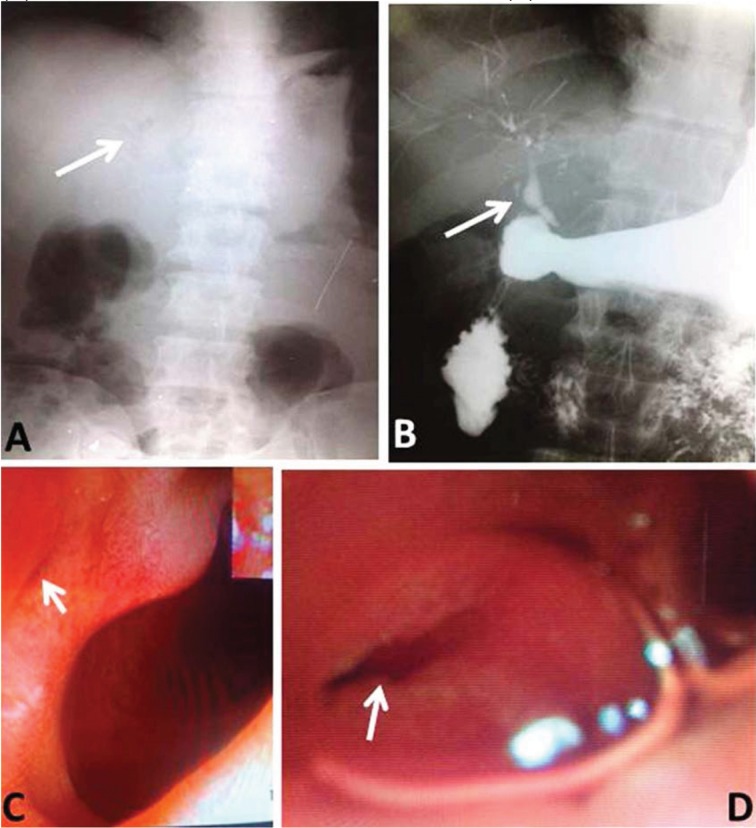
Choledochoduodenal fistula: plain x-ray of the abdomen showing pneumobila (A), water soluble contrast study showing contrast leak from duodenum into biliary tract (B), oesophagogastroduodenoscopy showing duodenal lumen and choledochoduodenal fistula (C), and closer view of choledochoduodenal fistula (D)
